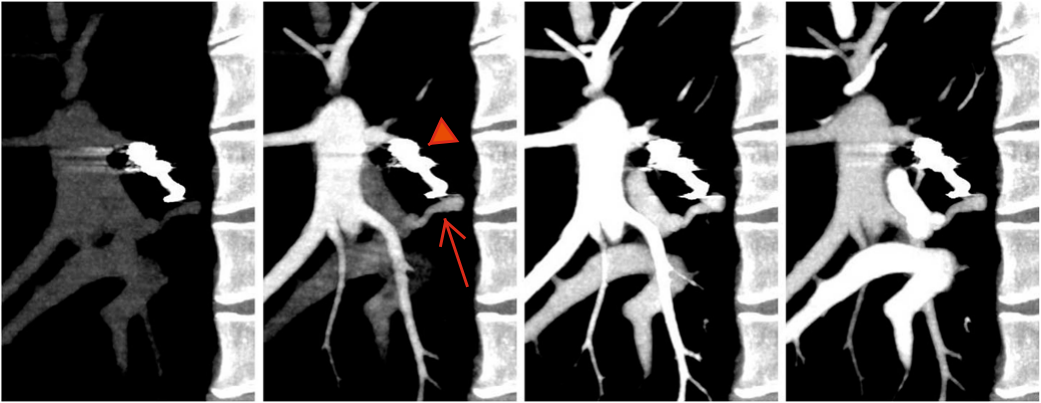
Dynamic CTA after the first intervention using dynamic volume scan with contrast enhancement. Early contrast filling and wash-out of aneurysmal sac of PAVF was noted. Enhancement of aneursymal sac was almost same as that of pulmonary artery (arrow) and suggested recanalization through the embolization coil (arrow head).
